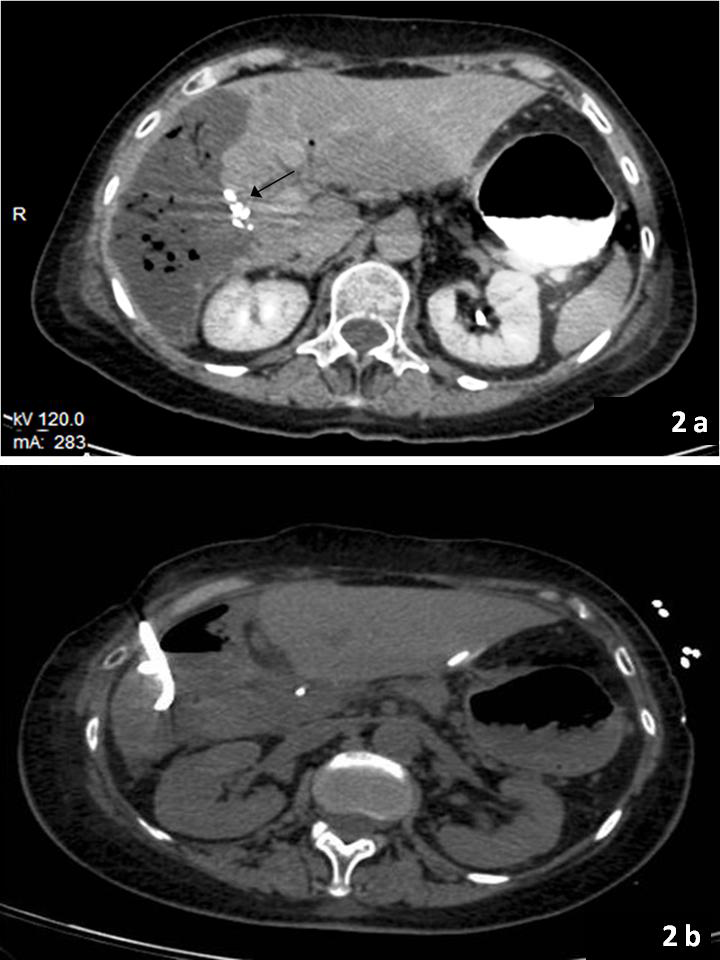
Post-operative Computed Tomography scan in case 2. a. Contrast-enhanced CT scan showed metal clips due to resection of liver segment V and VI (arrowhead) and infected lesion in VII and VIII liver segment (day 8). b. Percutaneous trans-hepatic drainage (day 10).
